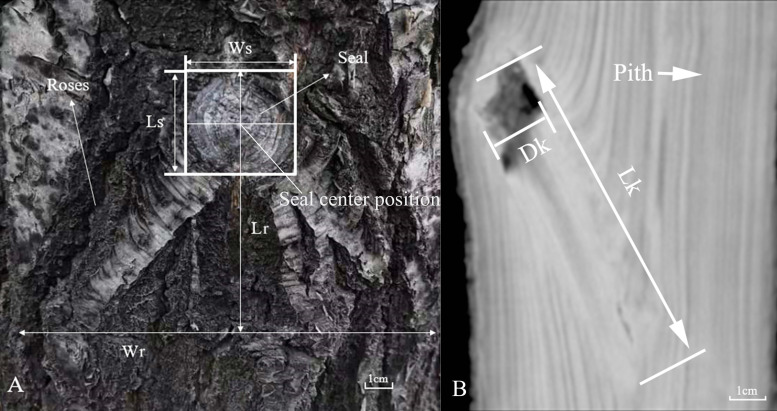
(A) Definition of scar (Ws, seal width; Ls, seal length; Wr, roses width; and Lr, roses length). (B) The occluded knot parameters on the CT image (Lk, knot length and Dk, knot diameter).
